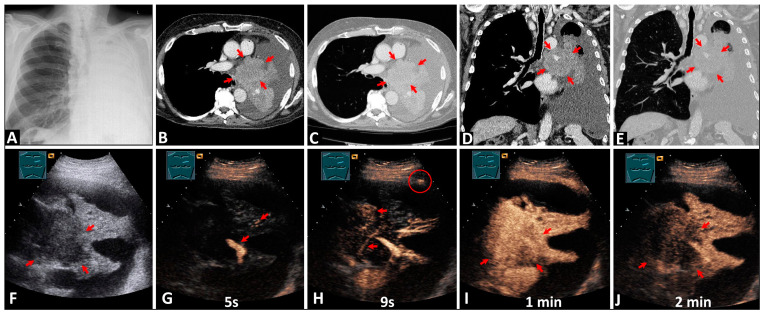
A 75-year-old patient with total opacity reduction in the left lung on chest X-ray (A) and suspected bronchogenic carcinoma. On computed tomography scans (B–E), a central tumor formation (arrows) with obstructive atelectasis is visible. On B-mode lung ultrasound (F), a central tumor (arrows) is distinguishable from the downstream atelectasis. On contrast-enhanced ultrasound, the atelectatic tissue shows inhomogeneous early pulmonary arterial enhancement (arrows) after 5 s, before the chest wall (G). The tumor tissue exhibits homogeneous delayed bronchial arterial enhancement (arrows) after 9 s, simultaneously with the chest wall (red circle), which is a sign of systemic vascularization (H). The tumor displays isoenhancement (arrows) compared with the atelectasis after 1 min (I), with rapid washout (arrows) after 2 min (J).
